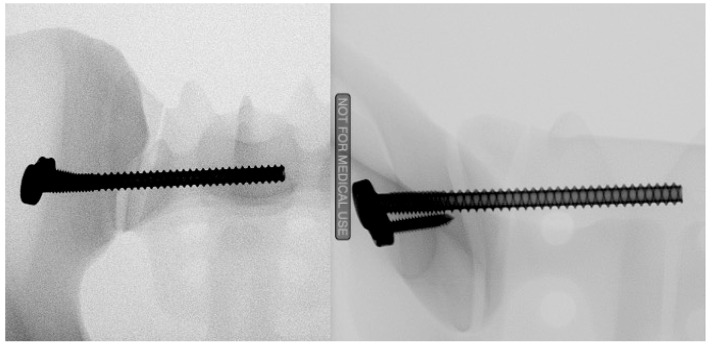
Radiograph of the SI plate fixation in two planes.
